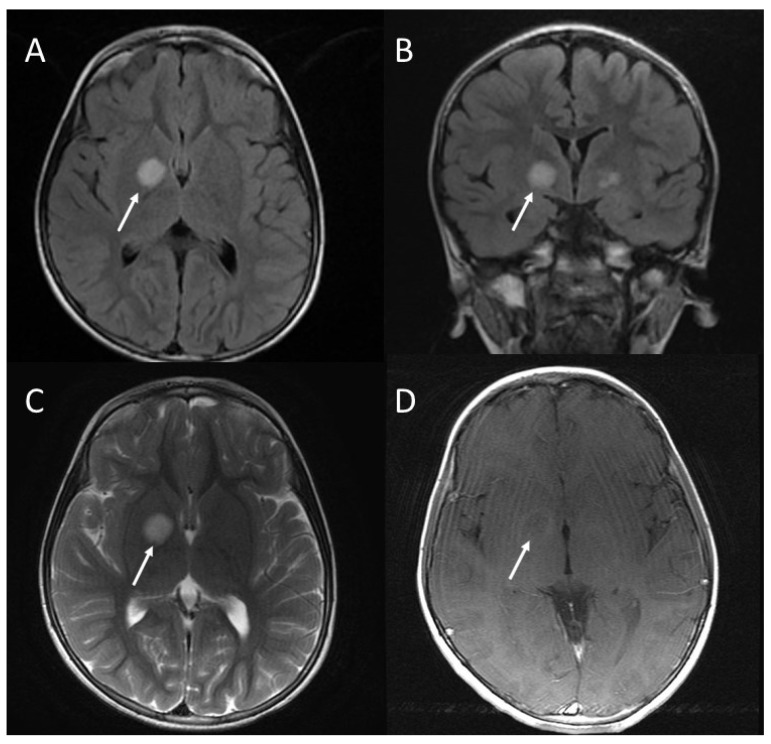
Focal area of signal intensity (FASI) in the right lenticular nucleus (arrows). Axial T2 FLAIR (A); coronal T2 FLAIR (B); axial T2-weighted FRFSE (C); axial T1-weighted FSE after gadolinium administration (D) [11].
